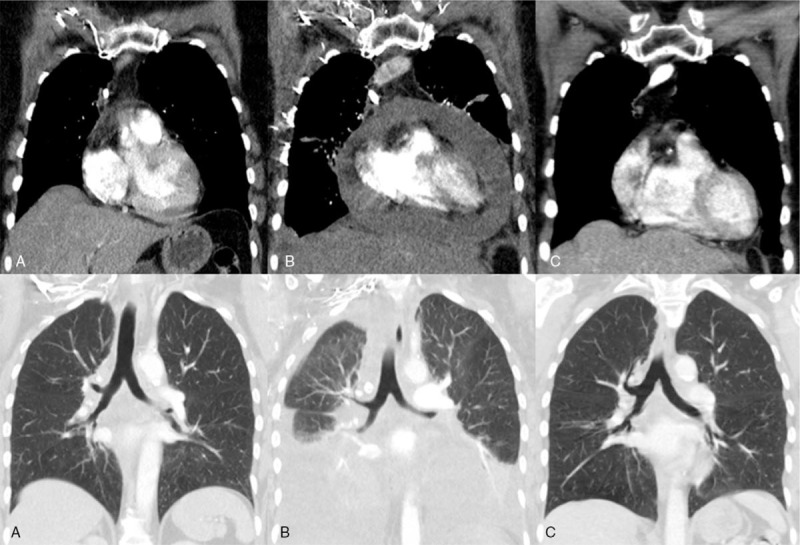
Chest CT scan of patient displaying hyperprogression top. Mediastinum view of pericardial effusion. Bottom. Lung view of pleural effusion. (A) Before pembrolizumab, (B) Hyperprogression, (C) Response to chemotherapy.
